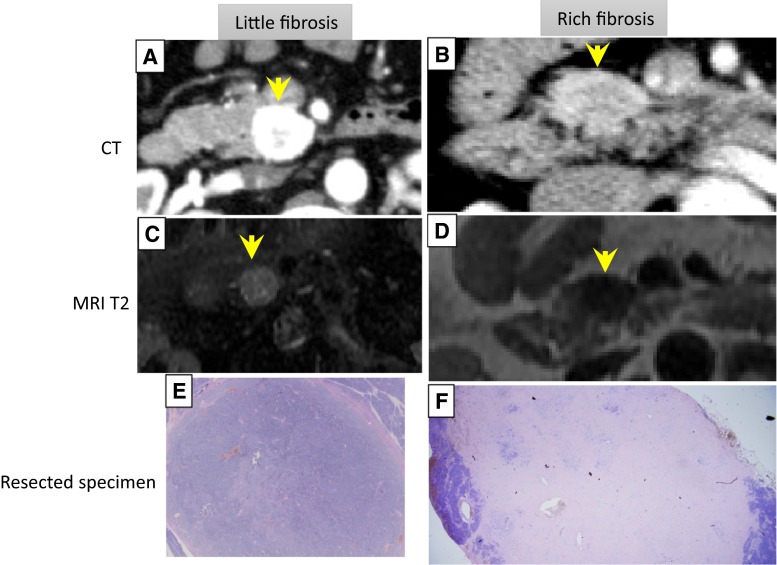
Representative cases of little and rich stromal fibrosis. a, c, e Images of pNEN with little fibrosis. b, d, f Images of pNEN with rich fibrosis. a, b CT shows strong and moderate hypervascularity in the tumor (*arrow*). c, d T2-weighted imaging shows a hyper- and hypointense mass in the pancreas head (*arrow*). e, f Low-power image showing weak and numerous fibrosis
